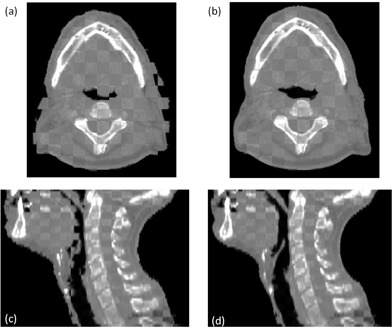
The checker board images of the pCT and CBCT from the second week of the treatment are shown in (a) and (c), respectively. The checker board images of the cCBCT (b) and CBCT (c).
